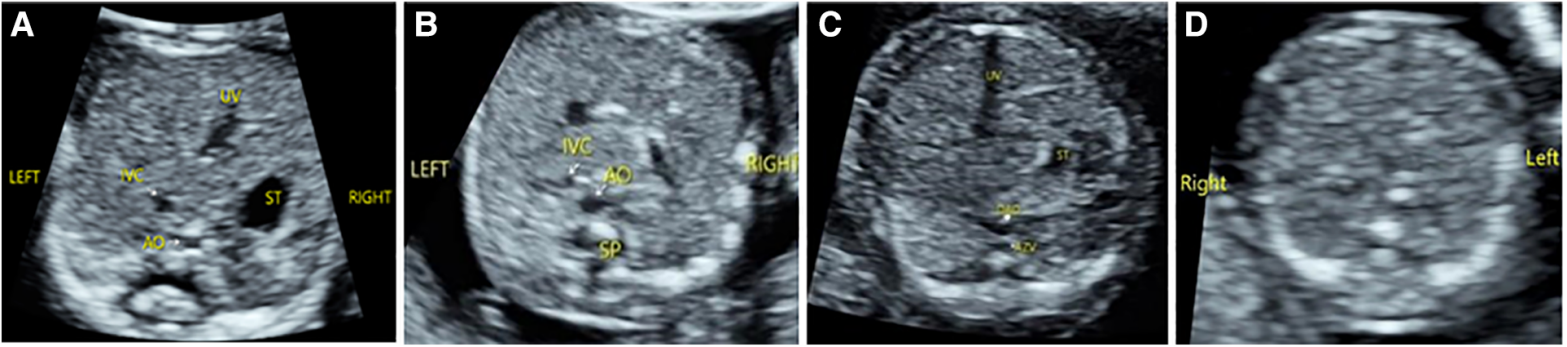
Abnormal image pattern of ultrasound in the upper abdominal transverse section. (A) Patterns of UAb-1: The stomach is on the right side of the abdomen, the superior vena cava is on the left front of the spine, and the abdominal aorta is on the right front of the spine. (B) Patterns of UAb-2: The stomach is small and located near the midline, while the superior vena cava is on the same side of the spine as the abdominal aorta. (C) Patterns of UAb-3: The stomach is small and located near the midline, and the dilated (hemiazygos vein) azygos vein is seen behind the right (left) of the descending aorta. (D) Patterns of UAb-4: The stomach is not seen on the transverse section of the upper abdomen.
